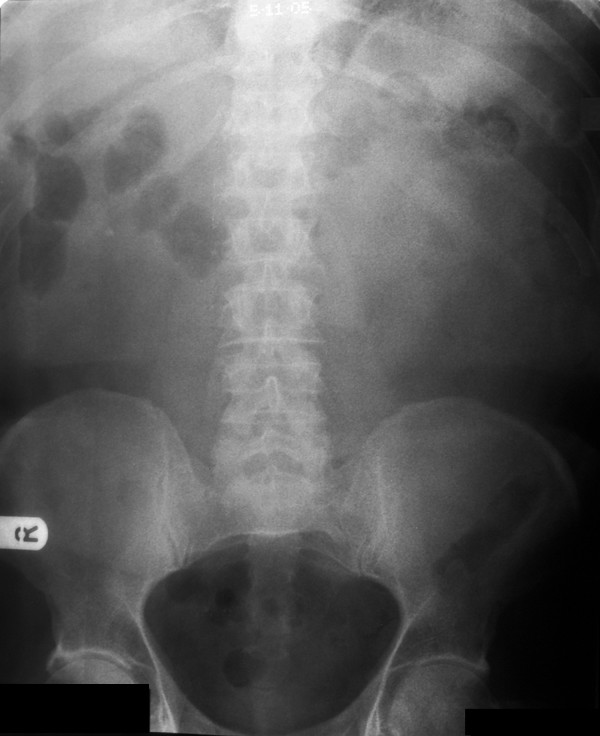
Abdominal radiograph showing no significant abnormality.
